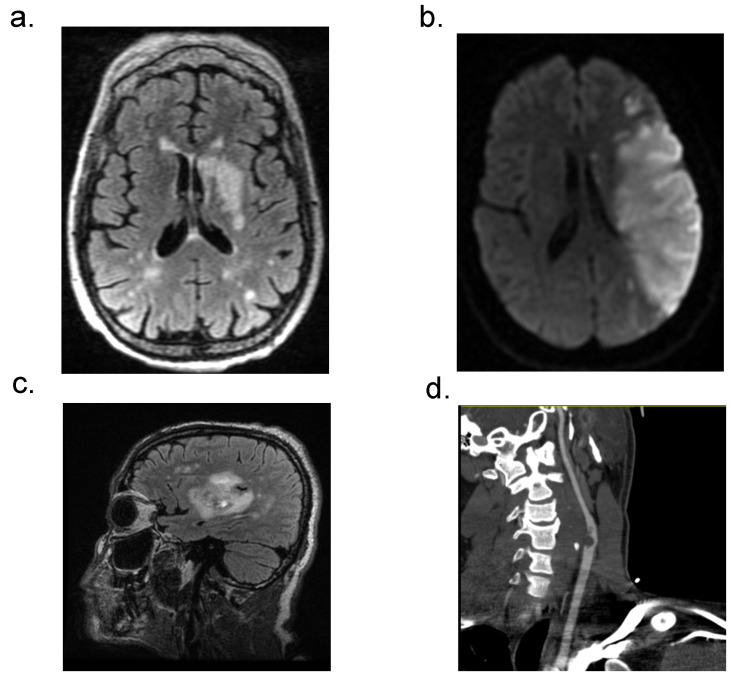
Neuroradiological manifestations of patients with CVD and acute COVID-19. (a) MRI FLAIR sequence with left basal ganglia and multifocal areas of ischemia. (b) MRI DWI image showing large left MCA territory infarct. (c) MRI FLAIR image of CVD in right basal ganglia (d) CTA image showing large clot in the internal carotid artery.
